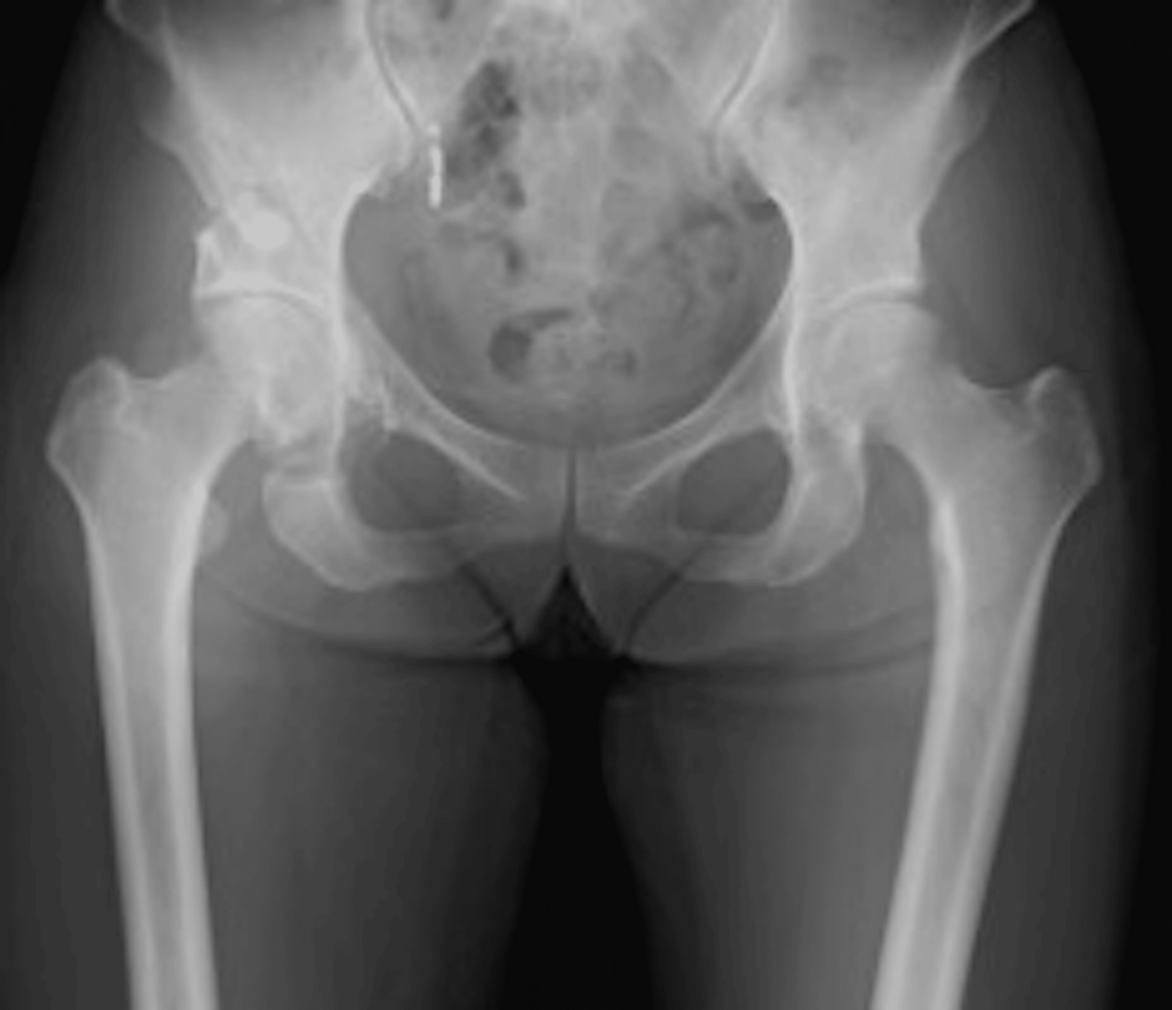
Postoperative radiographs of case 1. CE angle: 29.9°, ARO: 6.6°, Sharp's angle: 52.8°, AHI: 92.3%. CE, center–edge; ARO, acetabular roof obliquity; AHI, acetabular head index
